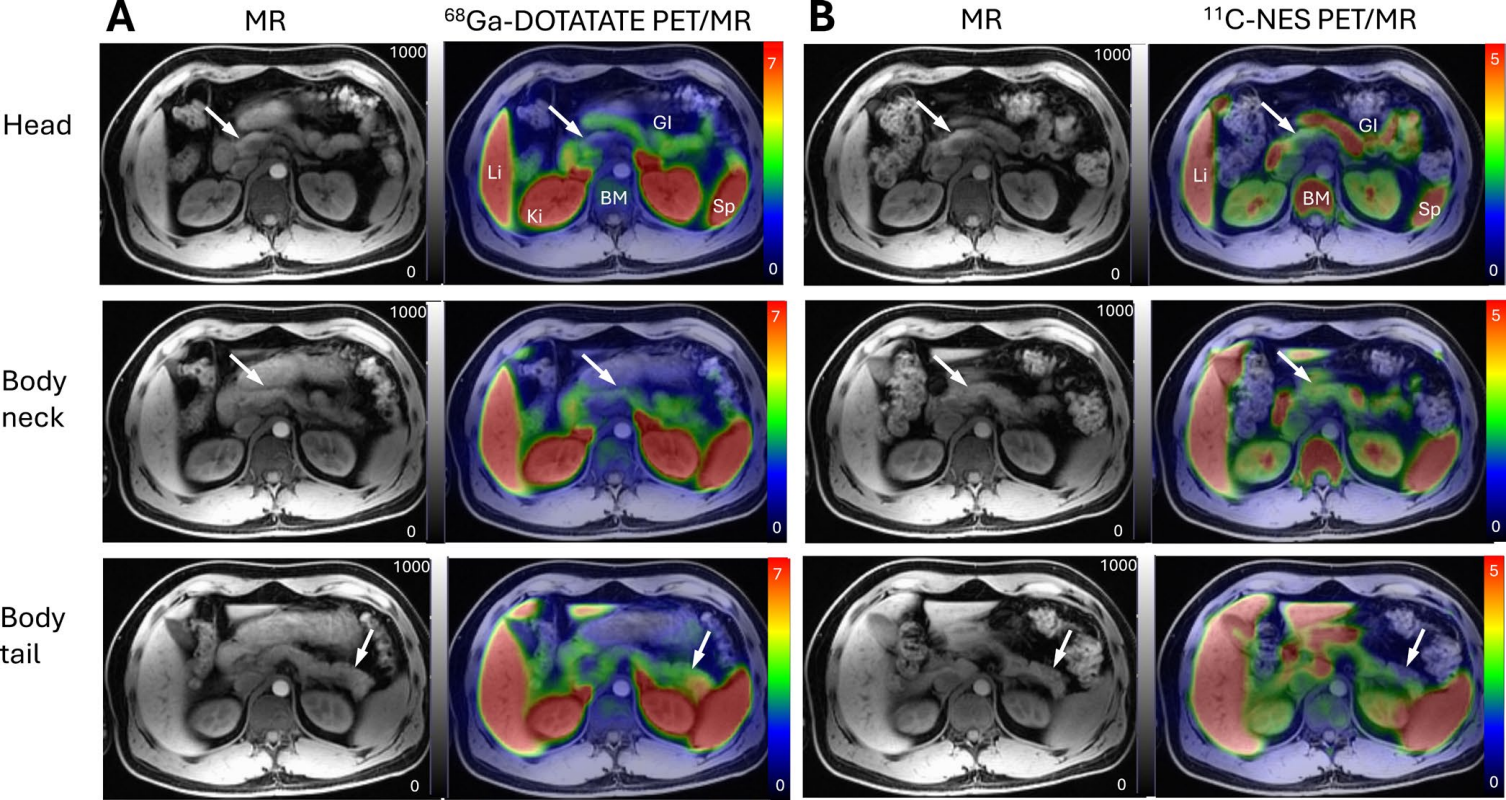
Representative PET/MRI images over the abdomen for the ^68^Ga‐DOTATATE and ^11^C‐NES PET‐tracers in a patient with long‐standing T1D. Both M1 macrophage marker ^68^Ga‐DOTATATE (A) and neutrophil marker ^11^C‐NES (B) demonstrated expected biodistribution and uptake in major abdominal organs. In pancreas, the binding of both ^68^Ga‐DOTATATE and ^11^C‐NES visually exhibited patchiness with some regions showing higher uptake and some regions having virtually no uptake. White arrows indicate pancreas on transaxial projections at the level of the pancreas head (caput), body (corpus) and tail (cauda).
